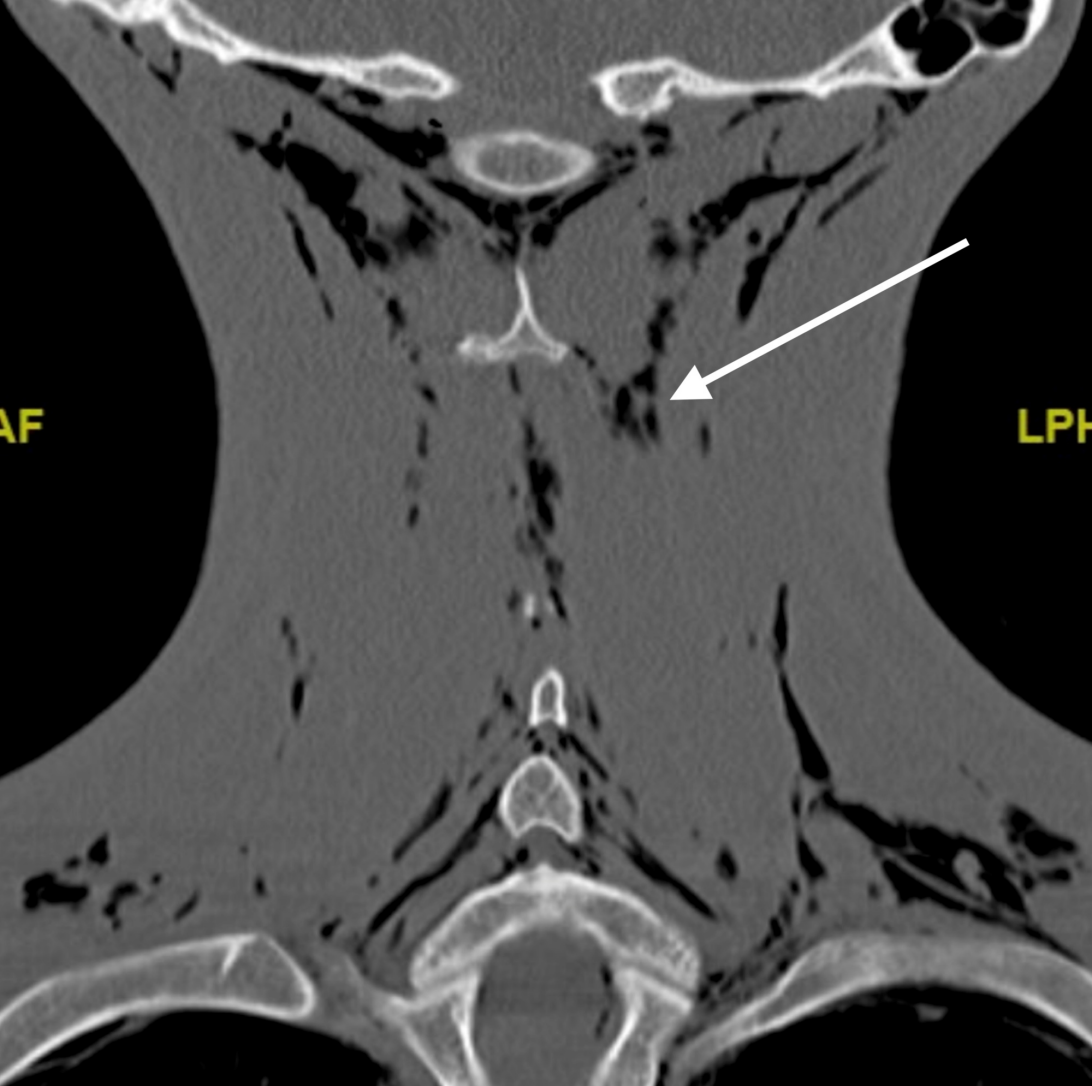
Coronal slice of cervical CT highlighting subcutaneous emphysema.
